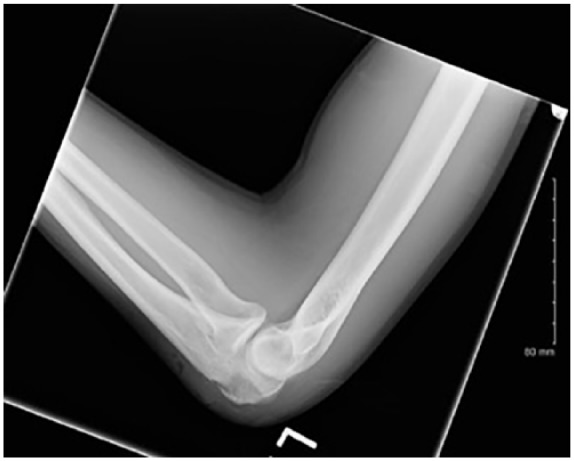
Left elbow X-ray on admission.
